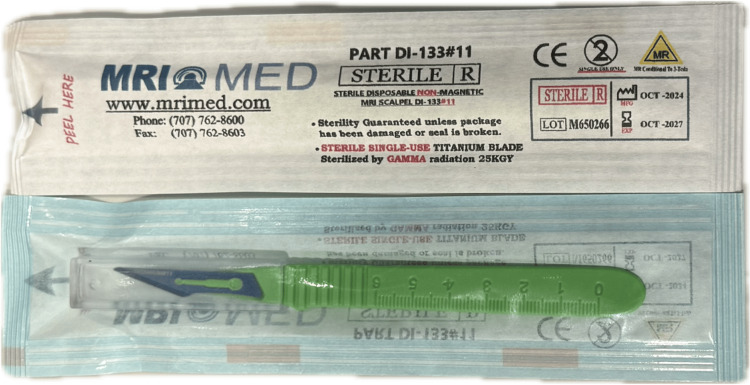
MR-conditional titanium scalpel (MRI Med, Petaluma, CA)
